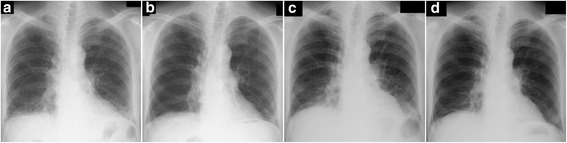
Changes of chest radiographs of patient #11. a The initial chest radiograph before treatment showed infiltration and volume loss of lower lung field. b After four months from the initiation of treatment, consolidation and volume loss improved. c However they arose again at the time of relapse. d At the end of follow-up, treatment improved infiltration and volume
